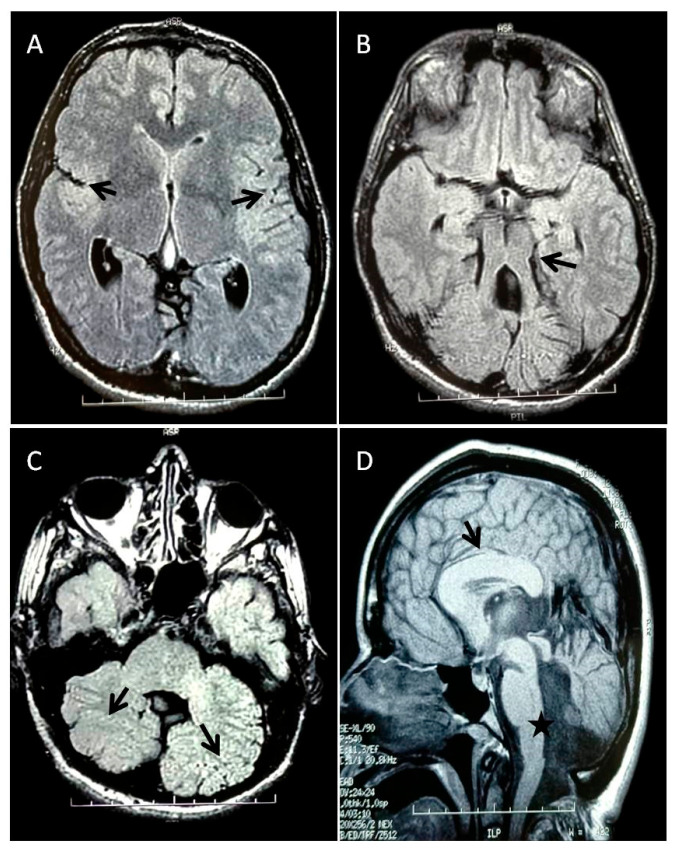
MRI brain images: T2 FLAIR axial images showing (A) reduced or absent opercularization (black arrows); (B) the molar tooth sign (black arrow) characterized by vermis hypoplasia, thickened cerebellar peduncles, and enlarged fourth ventricle; (C) diffusely abnormal foliation and fissuration of both cerebellar hemispheres (black arrows). FSE-XL sagittal image demonstrating (D) a thickened and stocky corpus callosum (black arrow) and an enlarged fourth ventricle communicating with a mega cisterna magna (black star).
